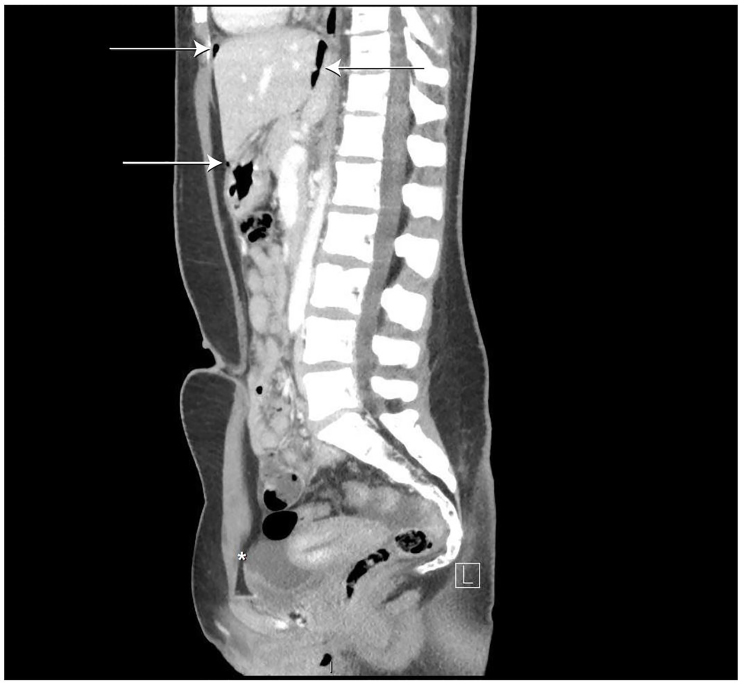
Sagittal CT image demonstrating free air (arrows) and free fluid (asterisk) in abdomen.
